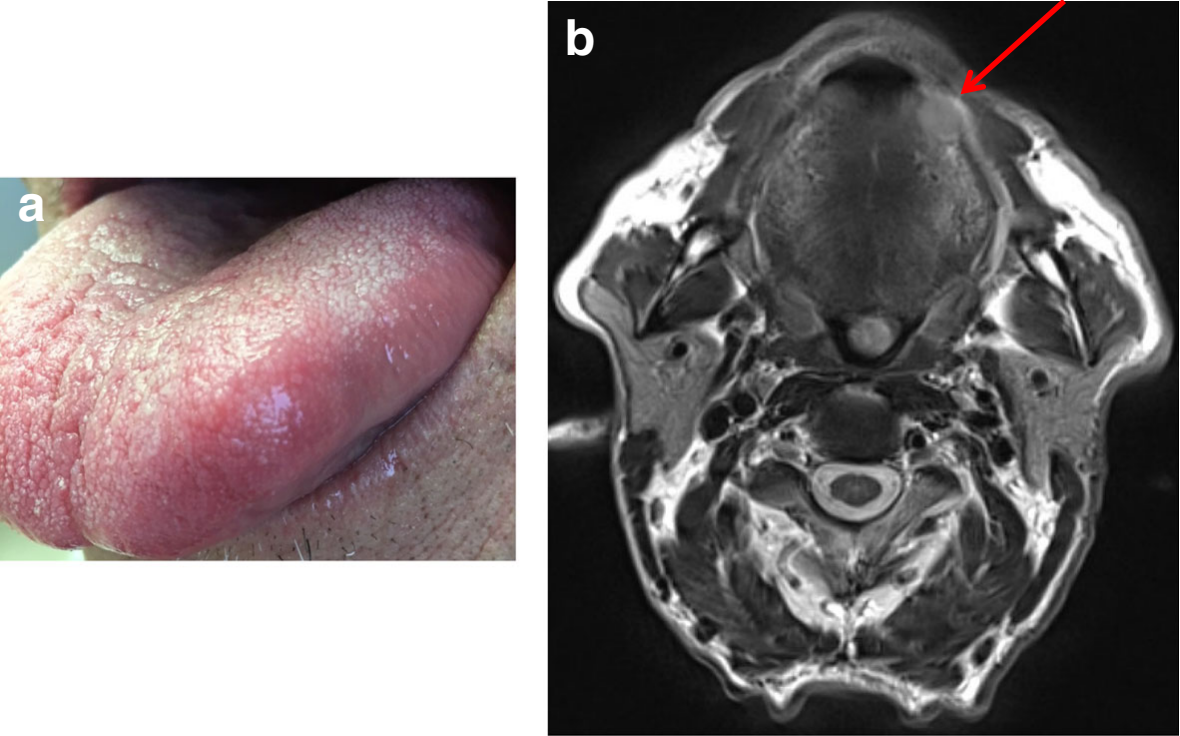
Lingual tumor localized on the left side of the tongue: clinical aspect (a) and MRI imaging (b) showing the 11 × 11 mm tumor mass
